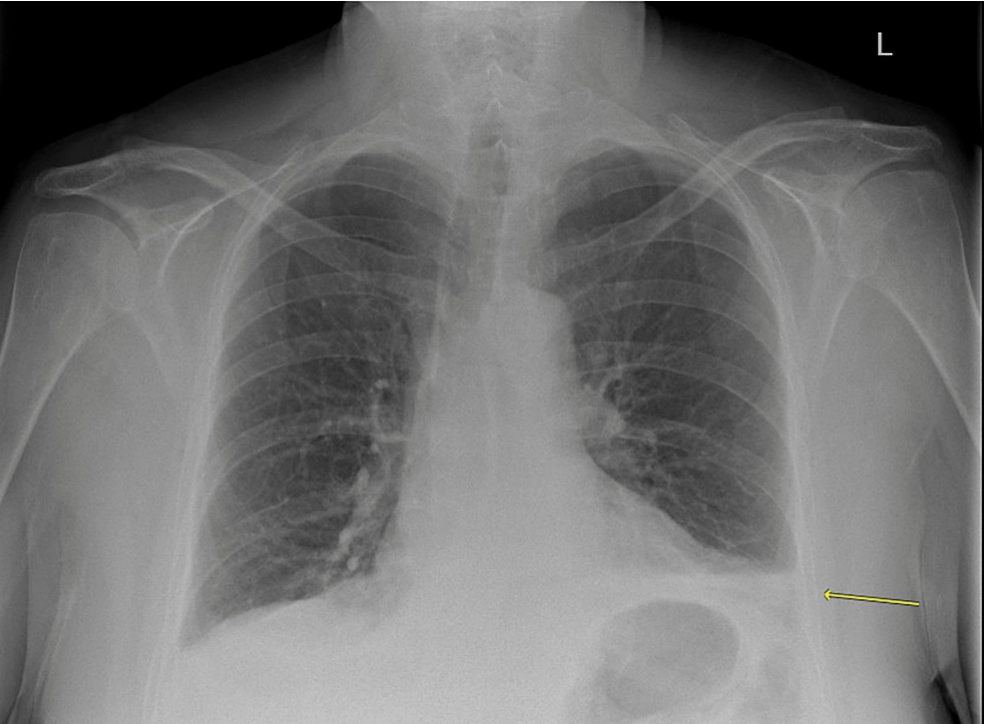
Chest X-ray showing left-sided pleural effusion, with an area of left lower lobe consolidation (yellow arrow) suggestive of Hampton’s hump infarct. The left lower lobe also shows regional oligaemia distal to the occluded vessel (consistent with Westermark sign suggestive of PE). PE: Pulmonary embolism
